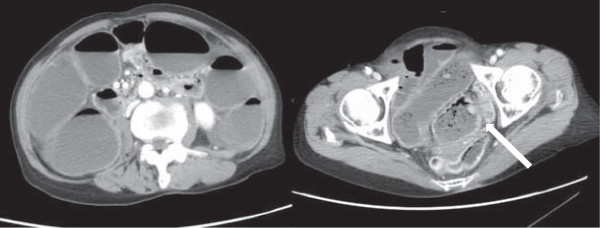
Abdominal enhanced computed tomography scan showing a large tumor in the sigmoid colon (arrow) and dilation of the colon and small intestine.
